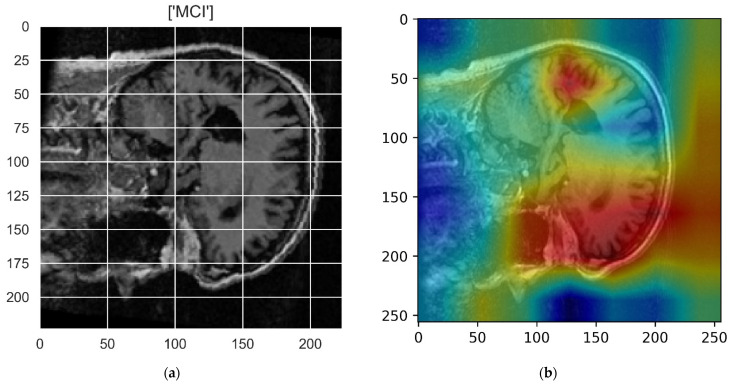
Grad-CAM explanation for the prediction of class MCI: (a) MRI image, (b) Grad-CAM attention map.
